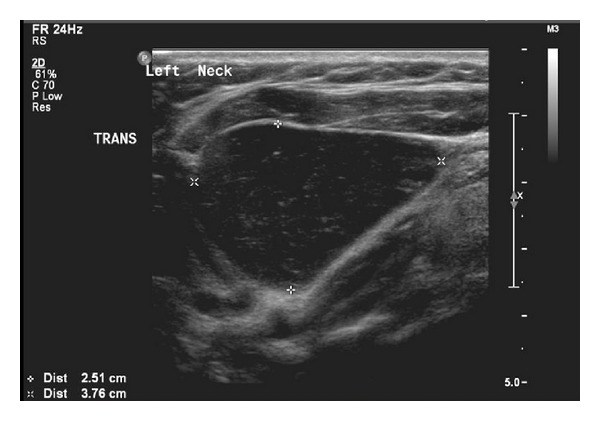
Ultrasound-transverse view showing the large cystic lesion.
